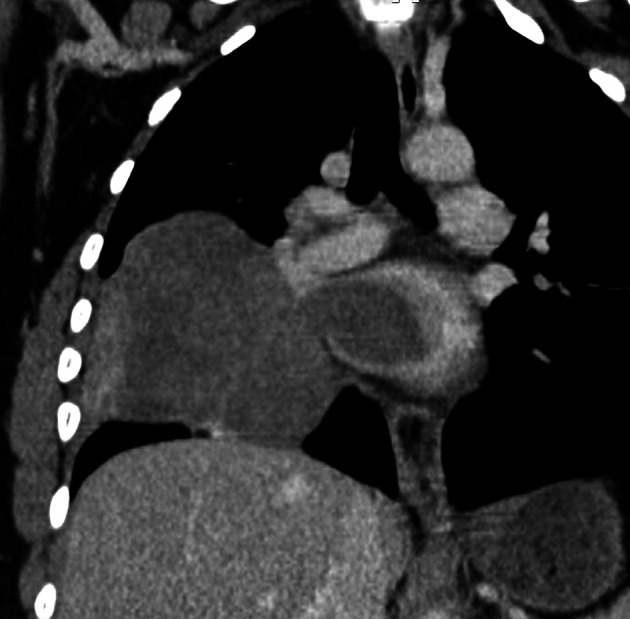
CT (coronal reconstruction): Right pulmonary mass, slightly enhanced after injection of contrast product with extension to the LA via the RIPV.
